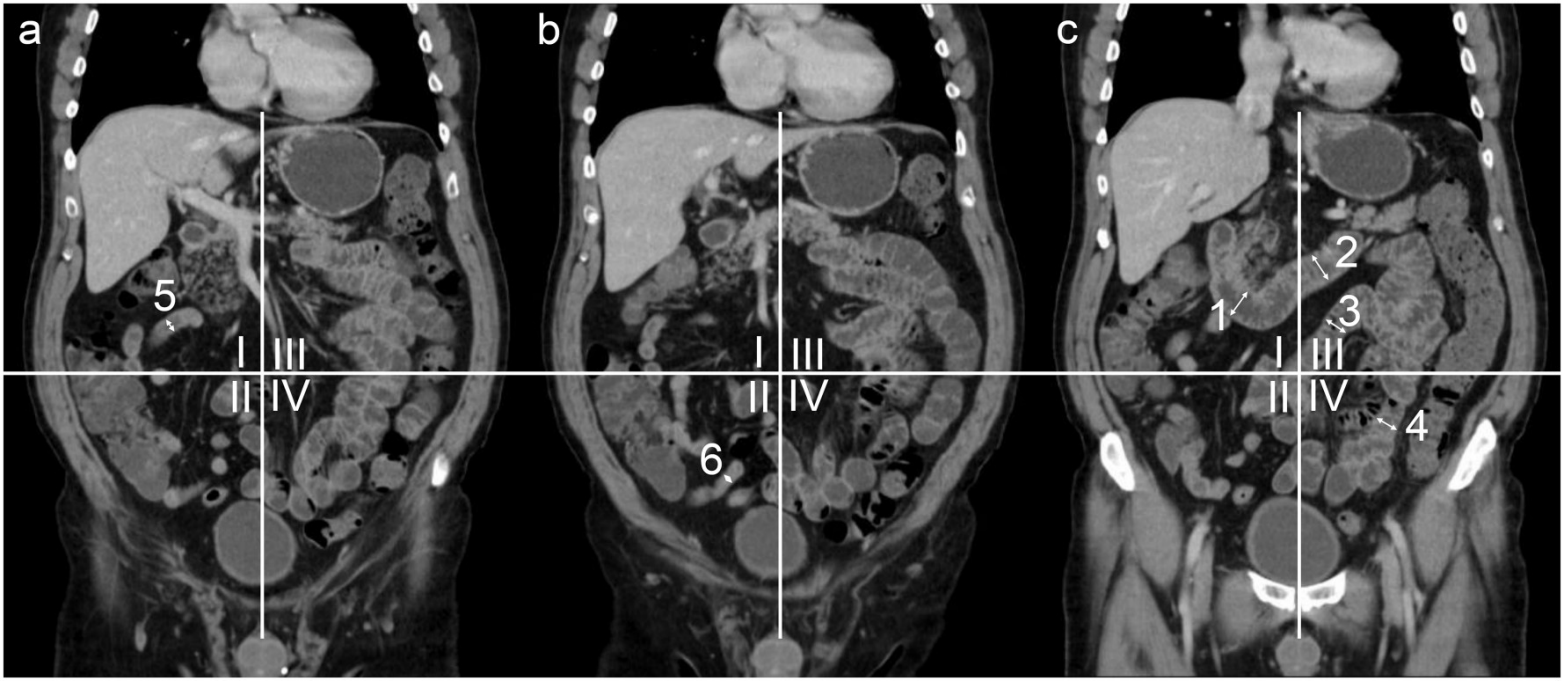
Coronal reformatted CT (three consecutive slices (a-c)) for demonstration of small bowel segment definition. The abdomen is divided into 4 quadrants with the umbilicus as the center point. Representative measurements of the descending (1) and horizontal duodenum (2). Jejunal and ileal segments were defined using the 4-quadrant model: I = upper right quadrant, including the proximal ileum (5); II = lower right quadrant, including the distal ileum (6); III = upper left quadrant, including the proximal jejunum (3); IV = lower left quadrant, including the distal jejunum (4).
